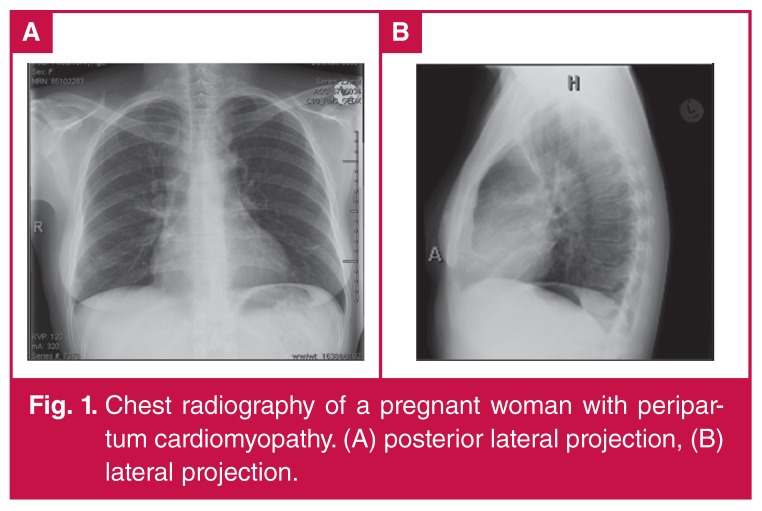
Chest radiography of a pregnant woman with peripartum cardiomyopathy. (A) posterior lateral projection, (B) lateral projection.
